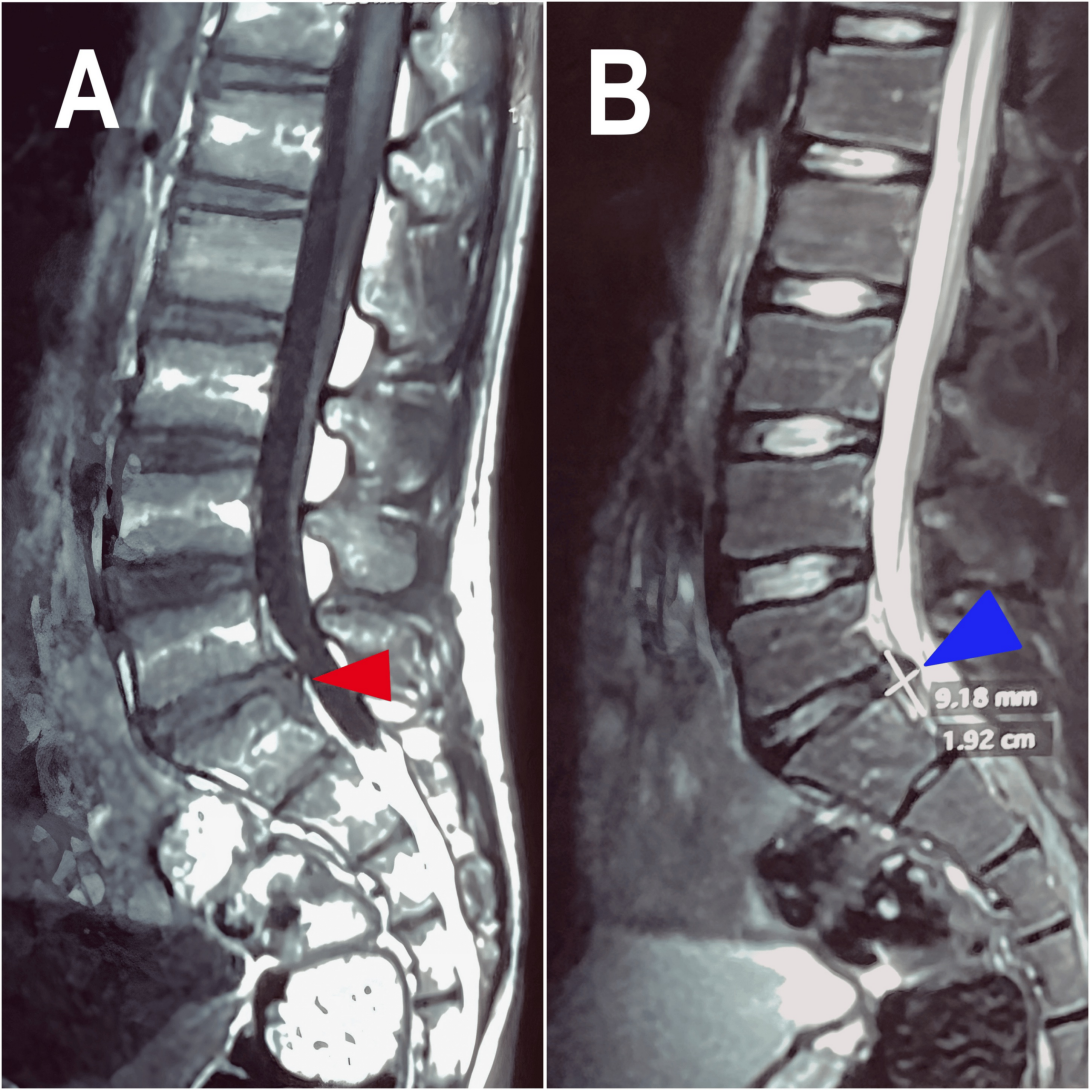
Sagittal T2-weighted MRI views of the lumbar spine (A) Suspected posterior ring apophyseal fracture at the S1 posterior vertebral body (red arrow), characterized by separation of a bone fragment at the posterior rim of the endplate, consistent with LARF. (B) Medium-sized central and paracentral disc extrusion at L5/S1 (blue arrow), measuring 1.9 cm in length, 0.9 cm in depth, and 2.5 cm in width. The extrusion results in moderate bilateral lateral recess narrowing with encroachment of both descending S1 nerve roots, as well as moderate bilateral exit foraminal narrowing without compression of the exiting L5 nerve roots. LARF, lumbar apophyseal ring fracture
